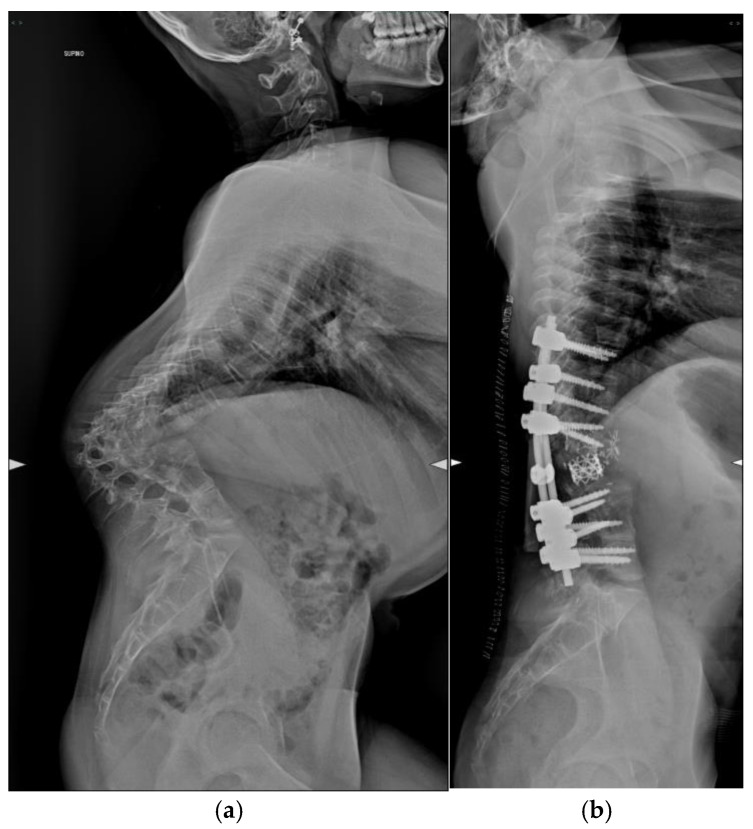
Lateral radiograph of Patient 3. (a) Preoperative assessment. (b) Postoperative assessment.
